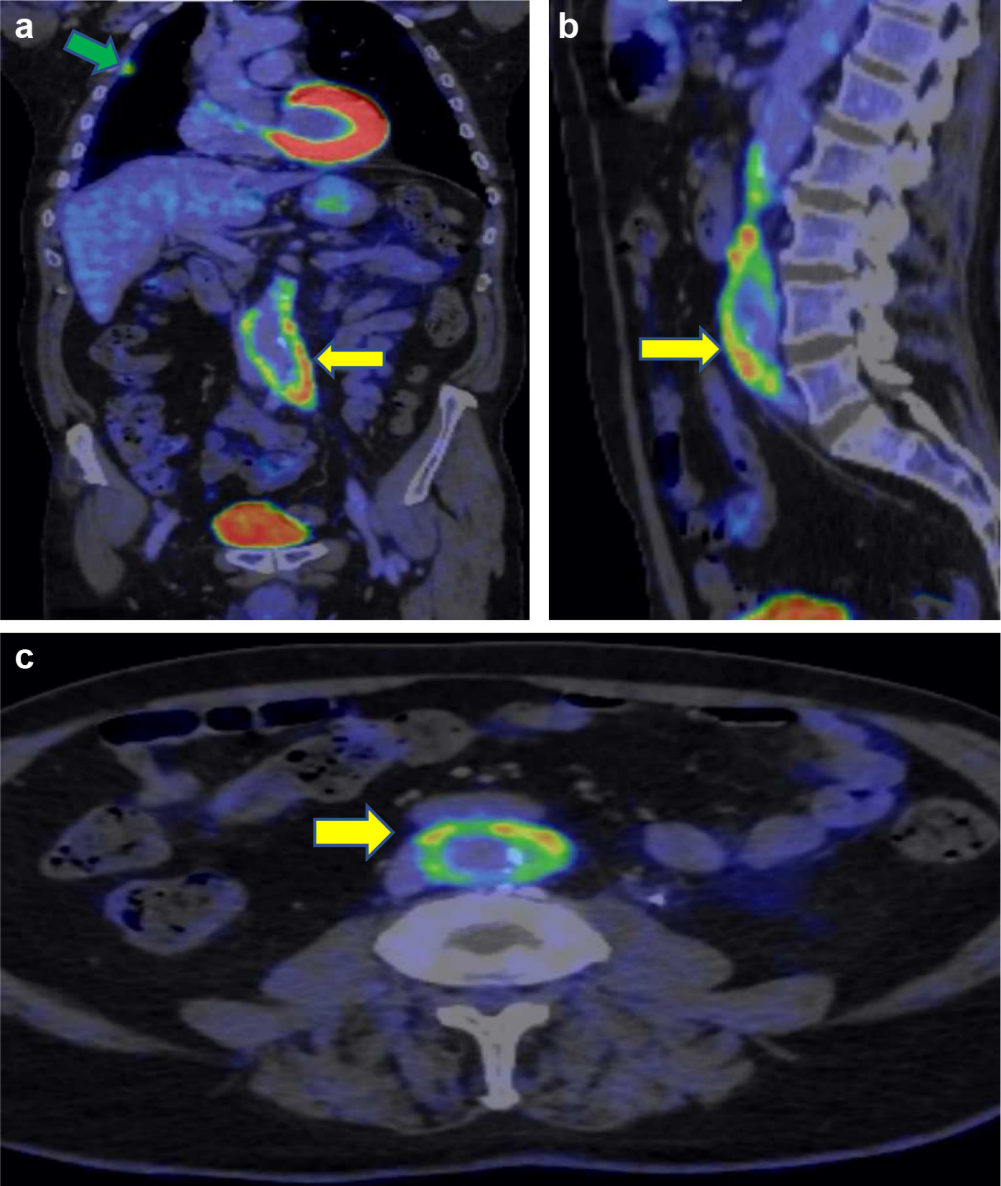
a-c, Positron emission tomography (PET)-computed tomography (CT) scans demonstrating extensive para-aortic soft tissue thickening with significant isotope uptake (*yellow arrows*), soft tissue thickening with increased uptake in the anterior mediastinum (*green arrow*).
